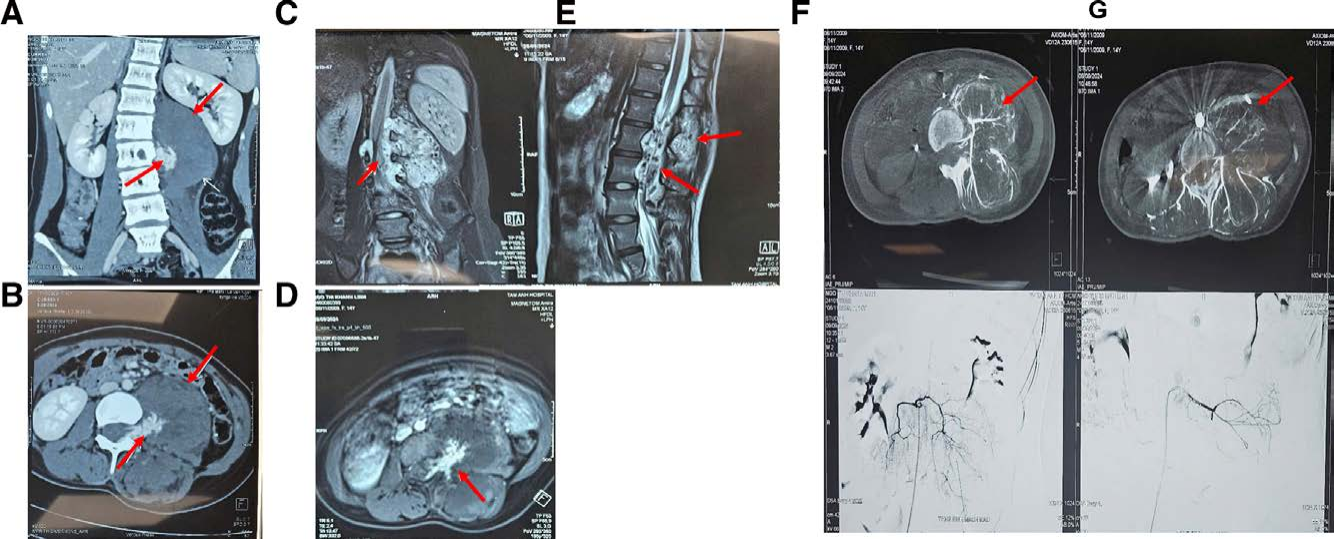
CT revealed a large vascular malformation in the iliopsoas muscle, measured 90 × 100 × 100 mm, in (A) coronal and (B) axial view. MRI revealed a large vascular malformation at the level of the T12 to L4 vertebrae in the iliopsoas muscle, compressed the conus medullaris and cauda equina, in (C) coronal, (D) axial, and (E) sagittal views. (F) Before and (G) after angiography and embolization of the vascular malformation, postintervention imaging revealed a hypervascular mass encompassed multiple paraspinal muscles and the left iliopsoas muscle, primarily fed by the left lumbar arteries at the L1 and L2 levels, underwent approximately 90% occlusion. The red arrow points at the hemangioma position. CT = computed tomography, MRI = magnetic resonance imaging.
